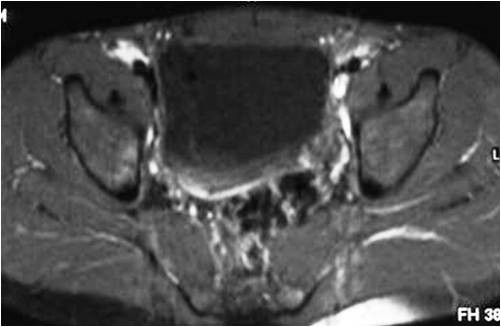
Follow-up MRI scan (6 months after antibiotic treatment initiation). On this axial spin-echo T1-weighted fat suppression with intravenous gadolinium contrast injection, image the right piriformis muscle has normal dimensions and signal intensity compared to the left piriformis muscle. There is no evidence of abnormal contrast medium uptake.
